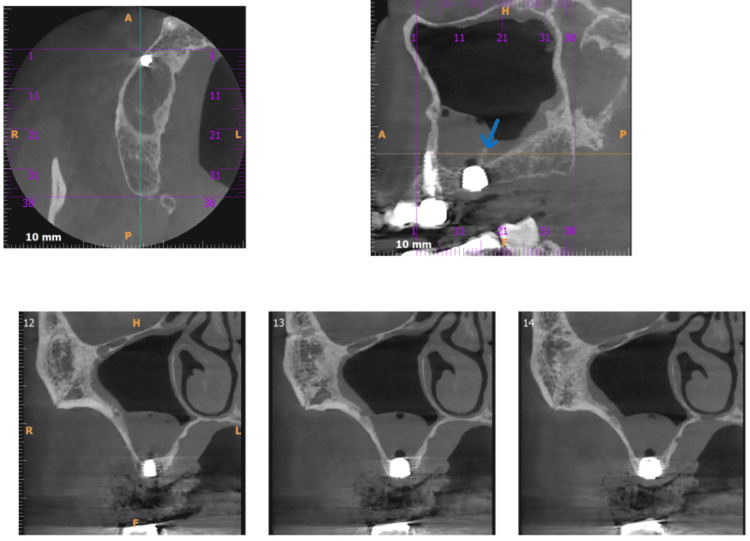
Immediate postoperative cone beam computed tomography after indirect sinus lift and short implant placement. The arrow shows blood pooling over the implant and bony fragments from the sinus lift procedure.
